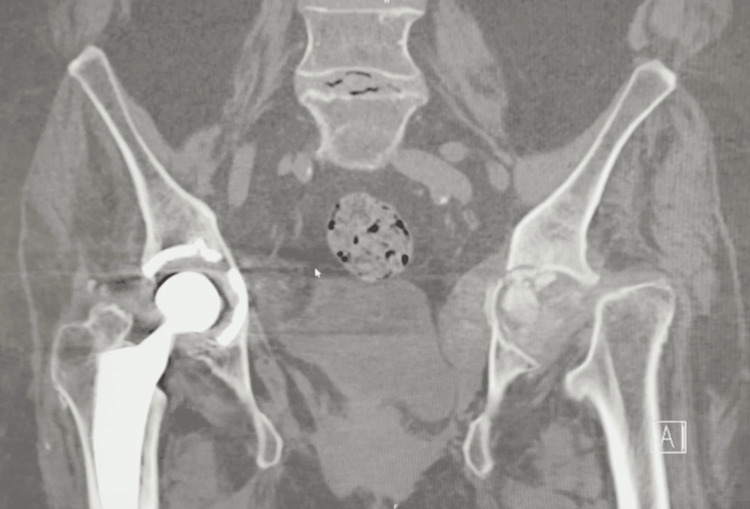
Preoperative coronal CT of the pelvis
